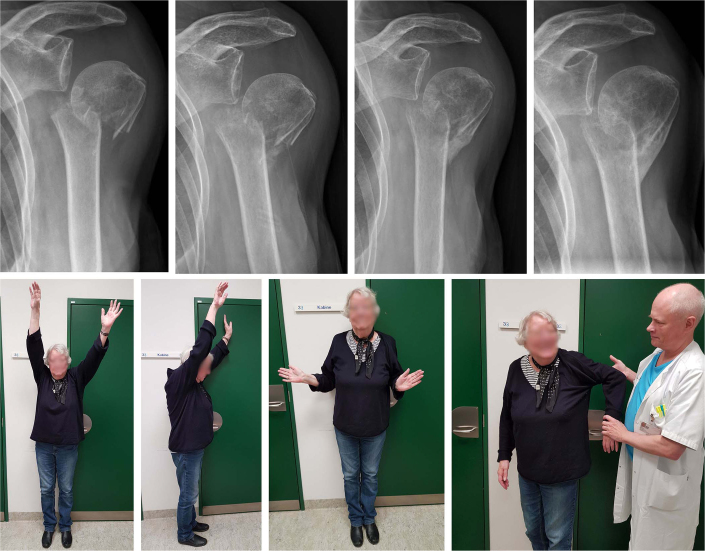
A 79-year-old healthy female with a 2-part surgical neck fracture, accompanied by medial translation of the humeral shaft. A lateral bone bridge was formed, allowing the patient to regain pain-free shoulder function. However, external rotation remained restricted. Radiographs at admission, 6 weeks, 12 weeks, and 24 weeks. Clinical photos at 24 weeks.
